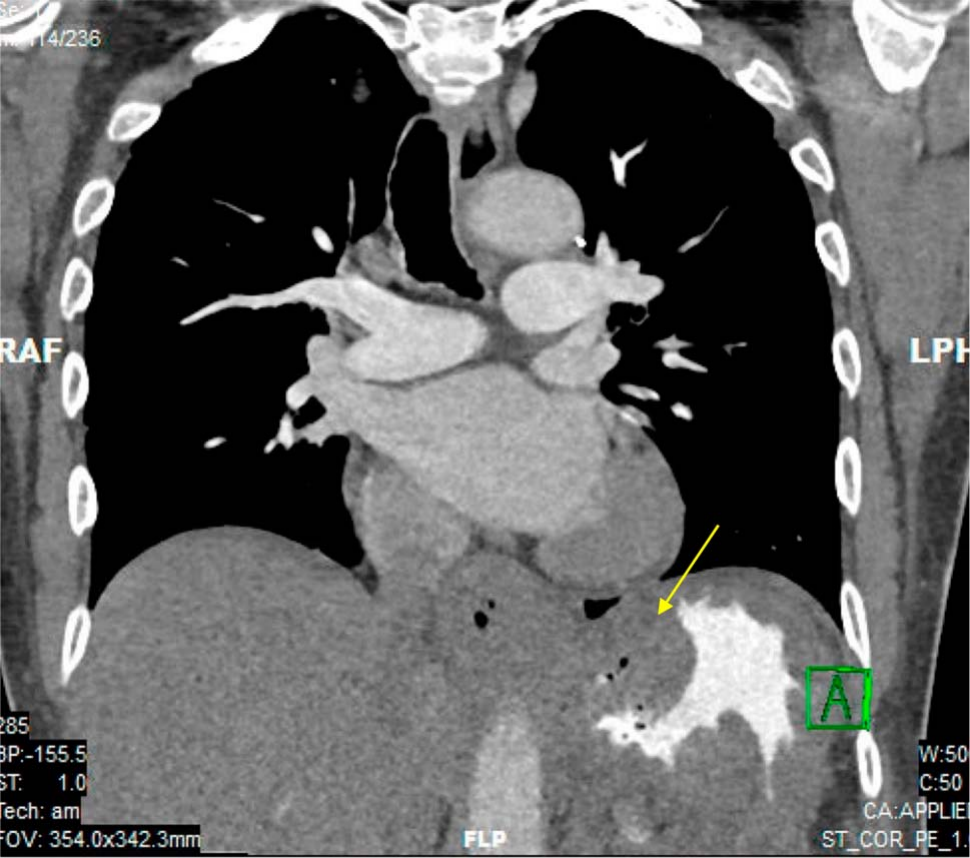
The computed tomography scan shows a thickened stomach with an infiltrating mass. This mass involves the distal esophagus, gastric cardia, and proximal stomach. The area of interest is highlighted by a yellow arrow.
